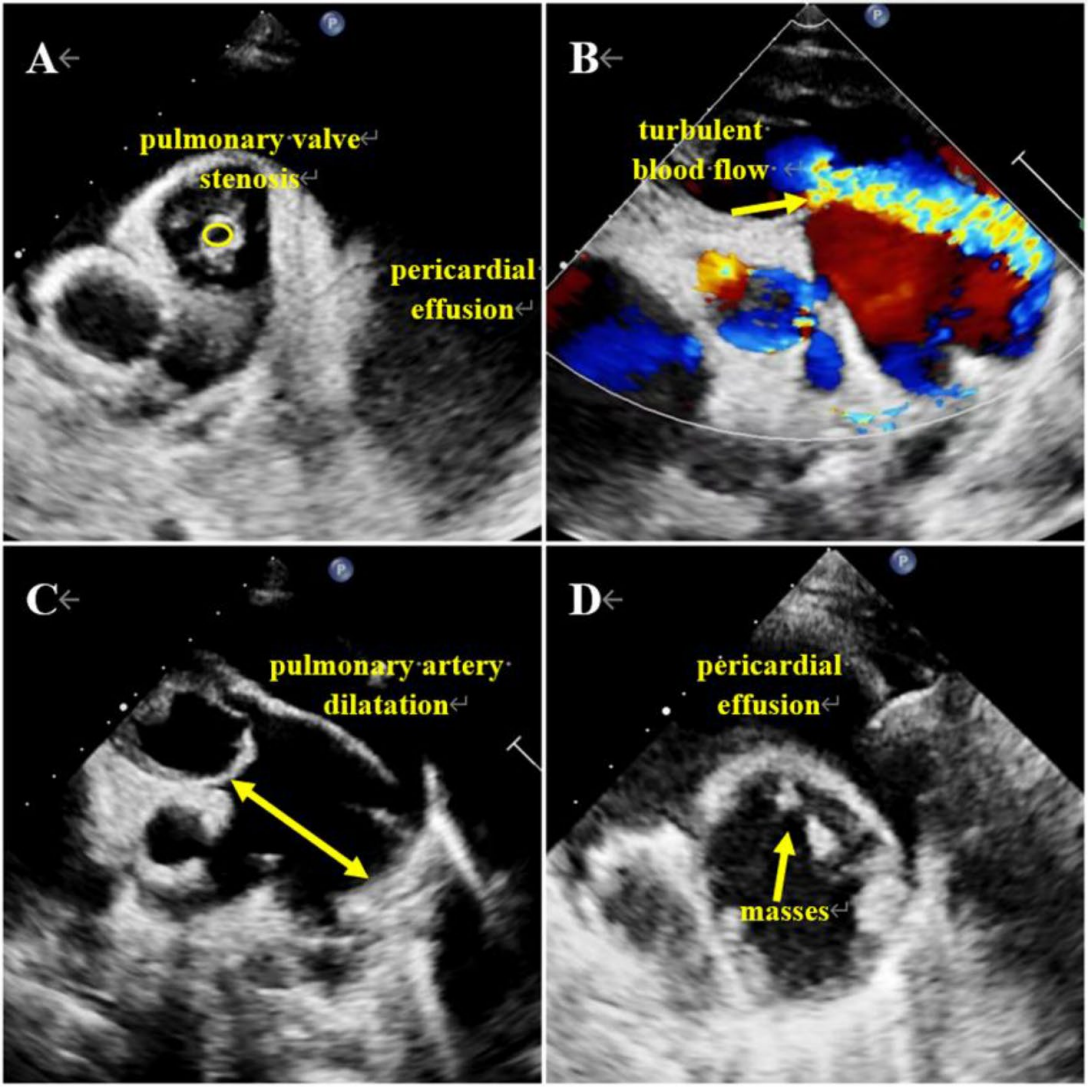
Echocardiography images of Case 2. (A) Pericardial effusion and pulmonary valve stenosis with an open area of 0.12 cm2 (oval). (B) Color doppler flow imaging shows that the turbulent blood flow signal in the pulmonary artery is brightly colored (arrow). (C) Pulmonary artery dilatation and trunk width of 4 cm (arrow). (D) Several masses in the dilated pulmonary artery (arrow)
